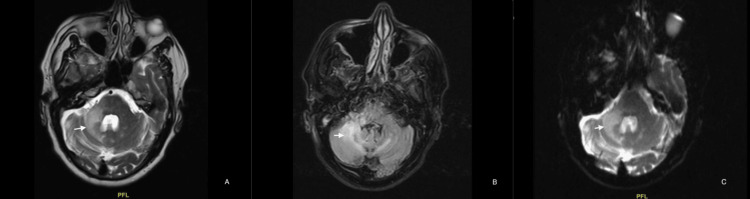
Follow-up MRI images (A) MRI brain with contrast shows hyperintensity (white arrow) in the right cerebellar hemisphere. (B) MRI brain FLAIR sequences show persistent hyperintensity (white arrow) in the right cerebellar hemisphere with a slight extension into the left cerebellar hemisphere as well. Although there were no interval radiological changes, the patient improved clinically. (C) MRI brain in the DWI sequence shows persistent hyperintensity (white arrow) in the right cerebellar hemisphere. FLAIR: fluid-attenuated inversion recovery; DWI: diffusion-weighted imaging
